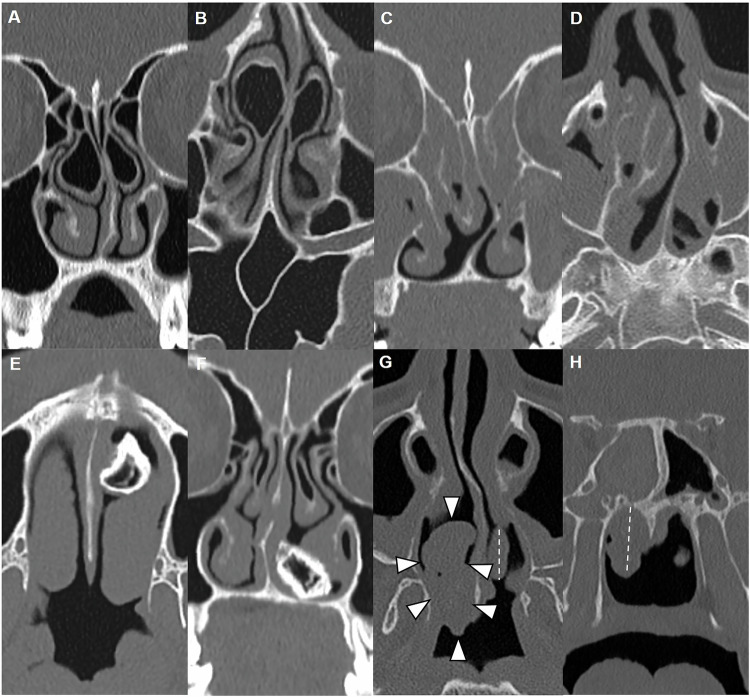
Overview of benign structural causes of nasal obstruction and epistaxis. Coronal (A, C, F, H) and axial (B, D, E, G) bone window sinonasal CT images. There is an enlarged and pneumatized middle turbinate on both sides with narrowing of the ostiomeatal complex (A, B). Left-convex nasal septal deviation (C, D) with architectural distortion and opacification of the ostiomeatal complex on both sides. There is also opacification of the maxillary sinus and the ethmoid air cells on both sides. A well-defined, rounded calcified lesion, consistent with a rhinolith (E, F), is noted between the left inferior meatus and nasal septum. There is a deviation of the nasal septum to the right. No evidence of bony destruction. Mucosal thickening also noted in the nasal fossa. Well-circumscribed nasal polyps (G, H) partially obliterating the posterior aspect of the right nasal cavity and protruding into the nasopharynx (white triangles in G) and a smaller lesion on the contralateral nasal cavity (dashed line in G). There are also findings of chronic sphenoid sinusitis on the right
